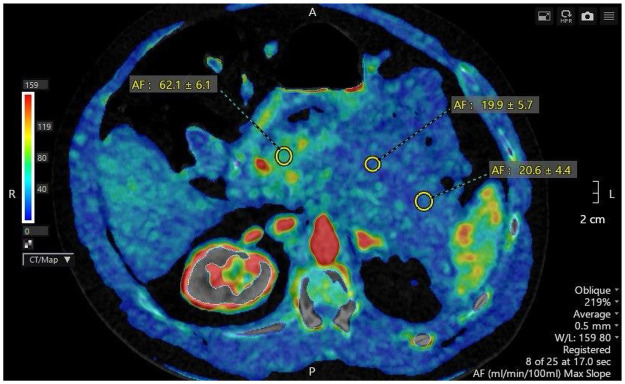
Perfusion CT of the pancreas.
